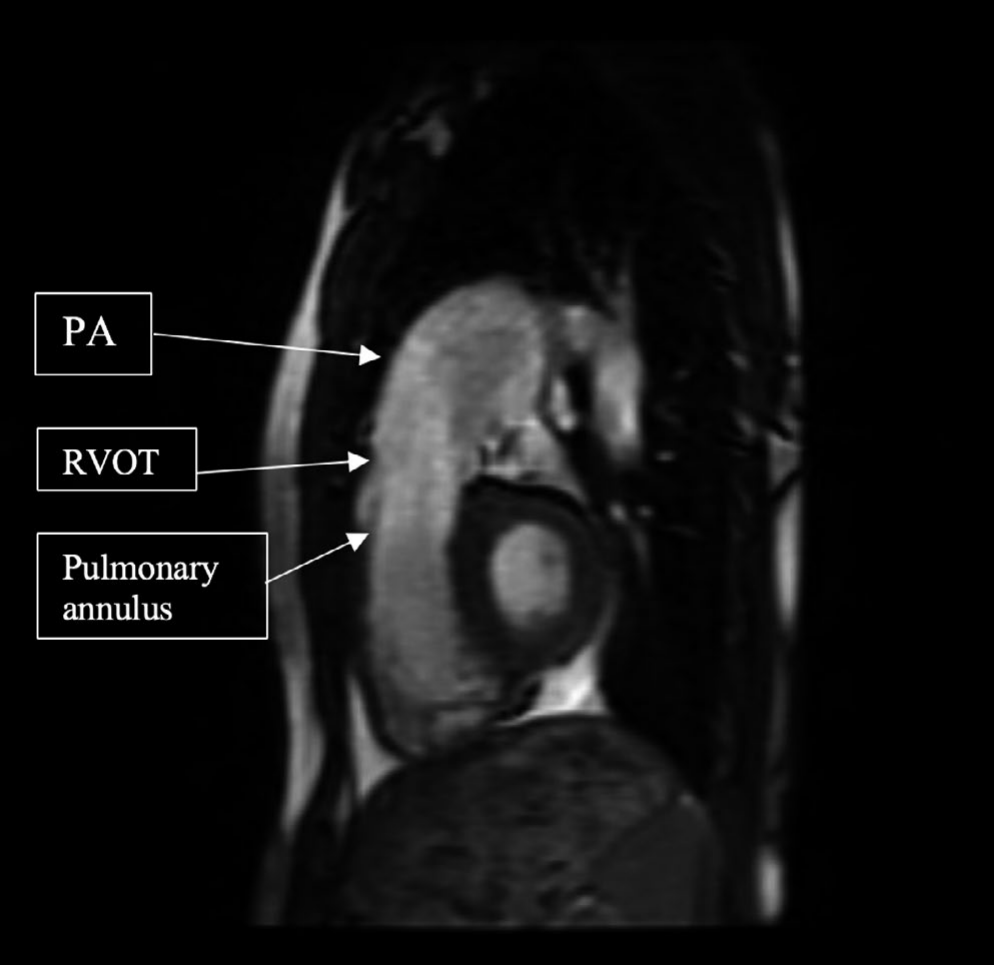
Sagittal right ventricular outflow tract (RVOT) view on cardiac MRI demonstrating the enlarged PA (43 × 41 mm), RVOT (27 × 35 mm) and pulmonary annulus (38 × 36 mm)
